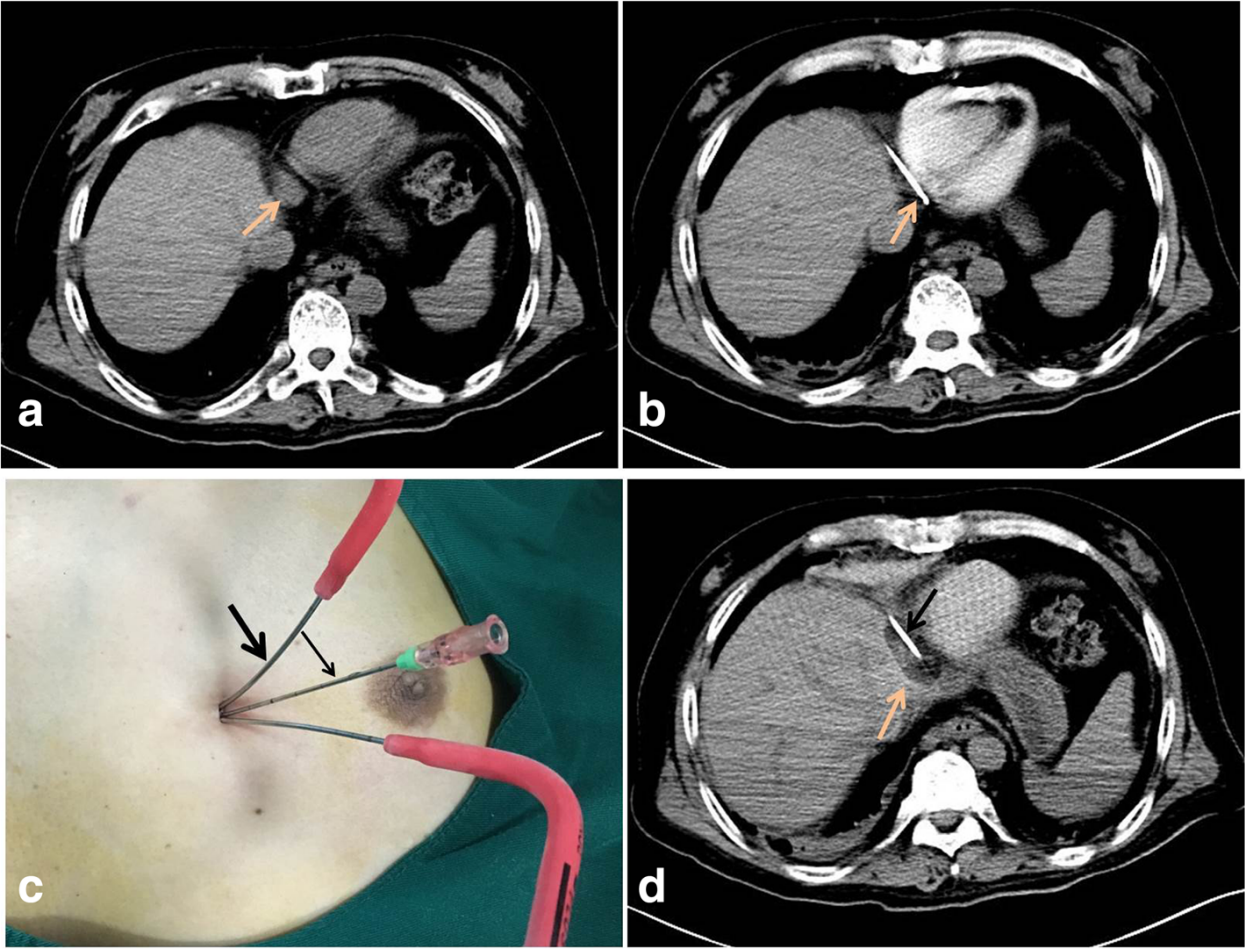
Intraoperative images of the patient. a Computed tomography image before cryoablation; the *arrow* indicates the tumor. b An 18G needle (*arrow*) was inserted into the pericardium and 200 mL of 0.9% saline solution was injected. c The *thick arrow* indicates the cryoprobes and the *thin arrow* indicates the 18G needle. d Computed tomography image of the ice ball (*pink arrow*) taken during the procedure to confirm that the ice ball had reached a sufficient size (3.5 × 3 × 2.8 cm^3^). The *pink arrow* indicates the cryoprobe
